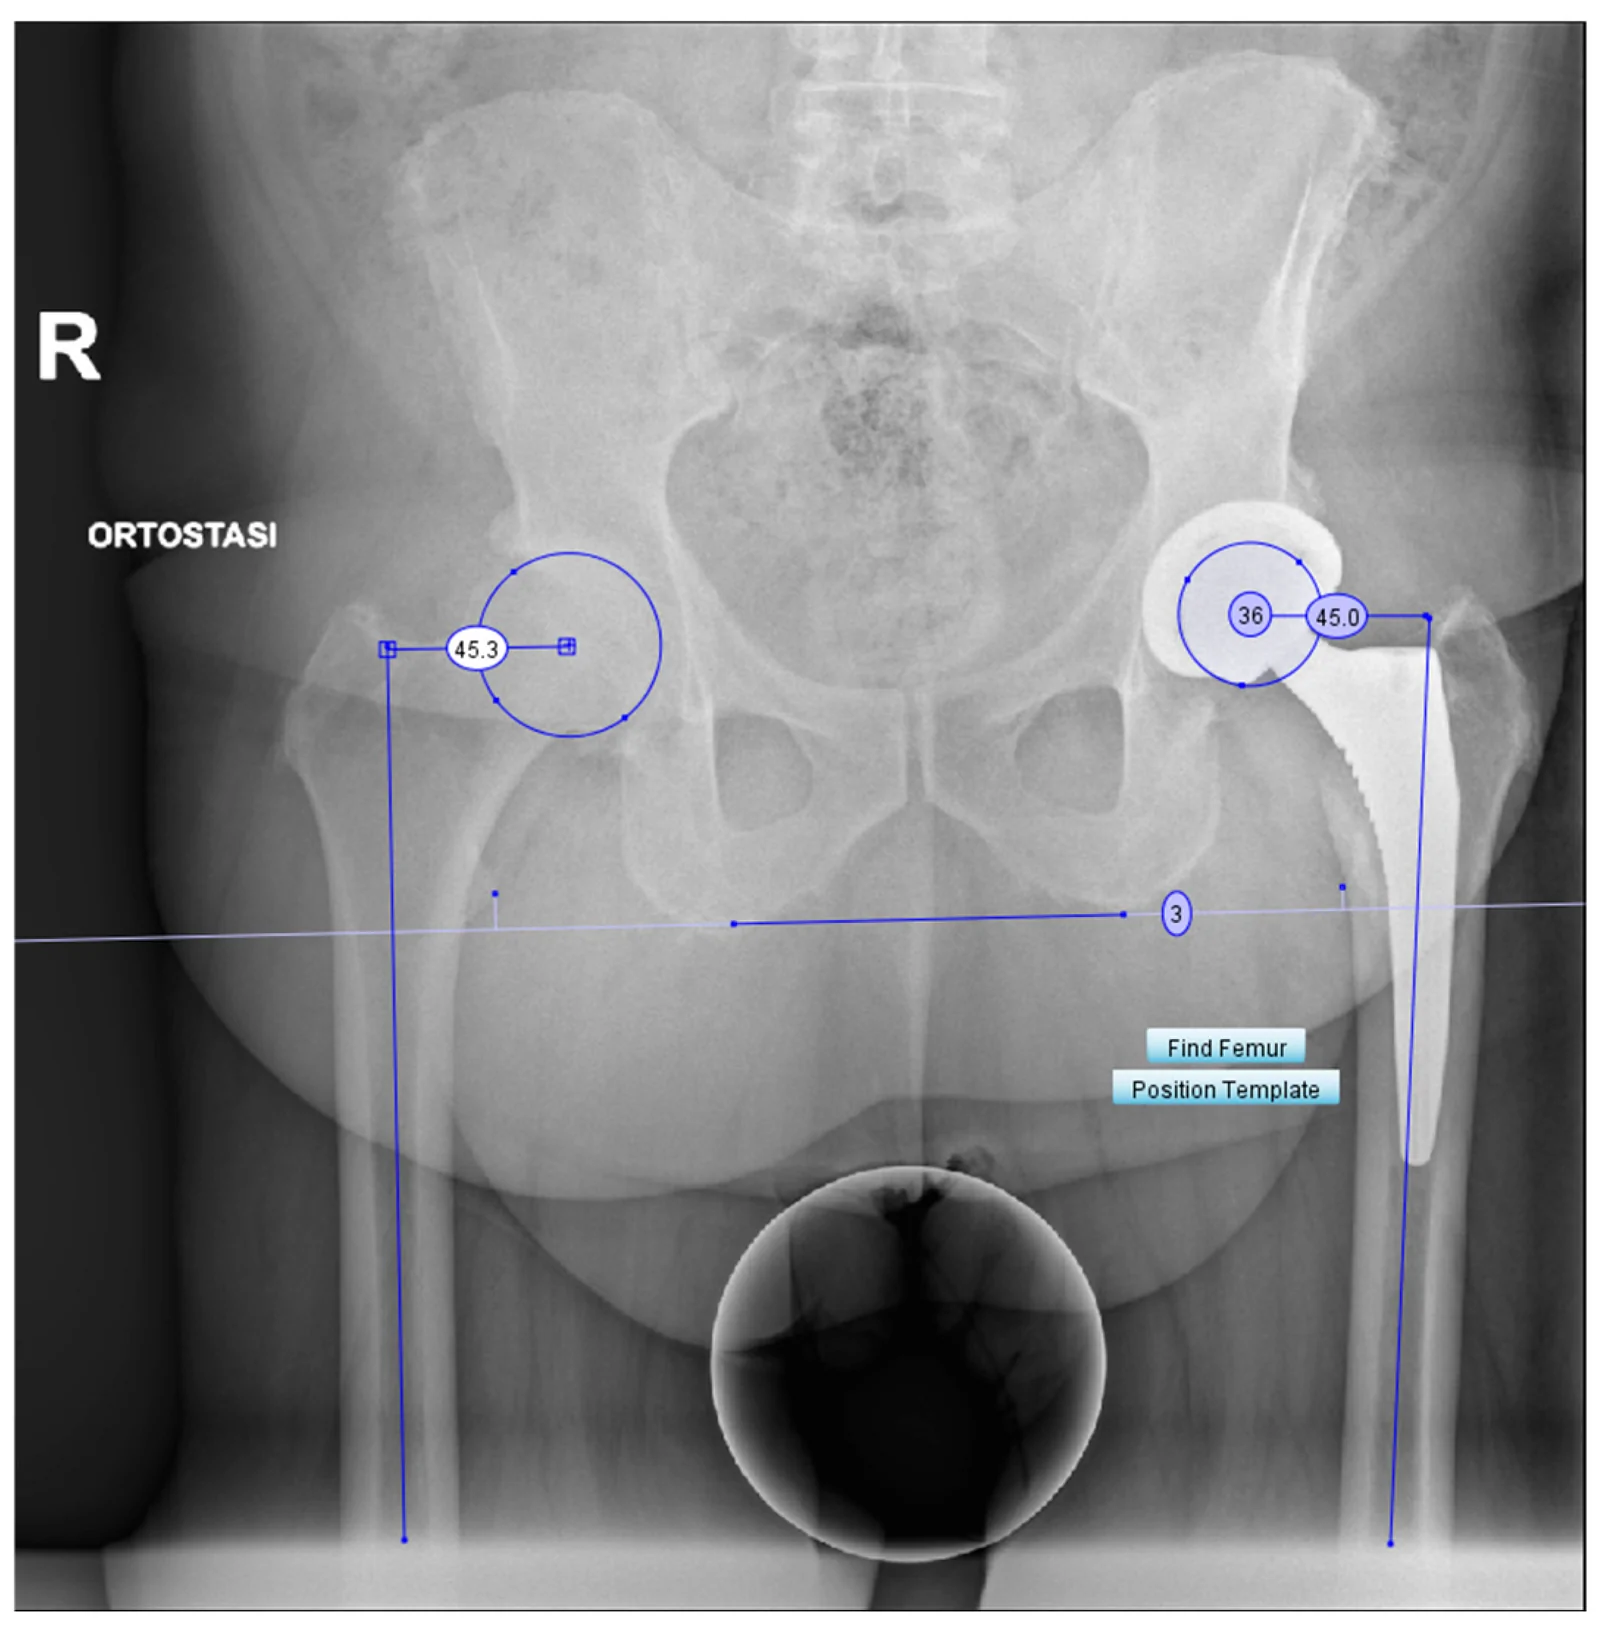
Pre-operative planning on an AP pelvis X-Ray performed in standing position: a horizontal line was drawn connecting the most inferior points of the ischial tuberosities. The vertical distance between this line and the most prominent point of the lesser tro-chanter was measured for each femur to assess the LLD; Femoral offset was defined as the perpendicular distance between the femoral head center and the femoral shaft axis.
